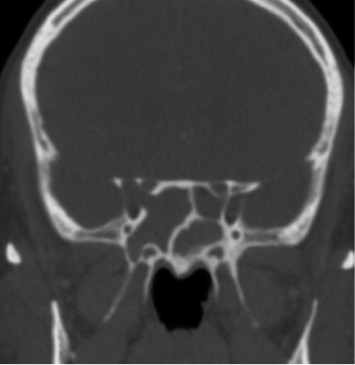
Computed tomography imaging showing diffuse opacification of the sinuses, with no skull base defect.
